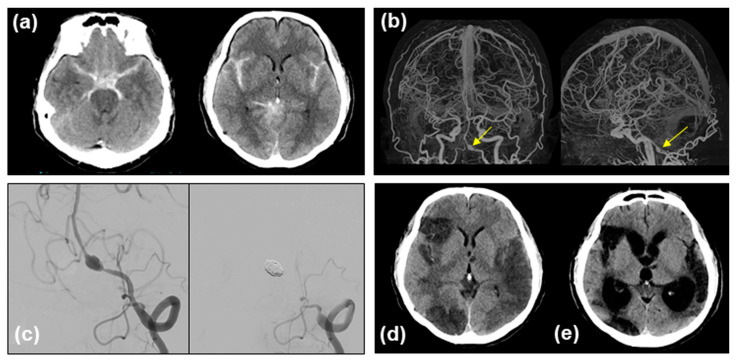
Imaging findings. Initial brain CT scan (a) and CT angiography (b). A left VADA detected by CT angiography ((b) arrows: (left), anteroposterior projection; (right), left lateral projection) was successfully treated by internal coil trapping. (c) DSA before (left) and after (right) the treatment. Follow-up CT scans on day 14 (d) and day 90 (e) suggest multiple vasospasm-related infarctions in bilateral MCA regions and the occurrence of hydrocephalus, respectively.
